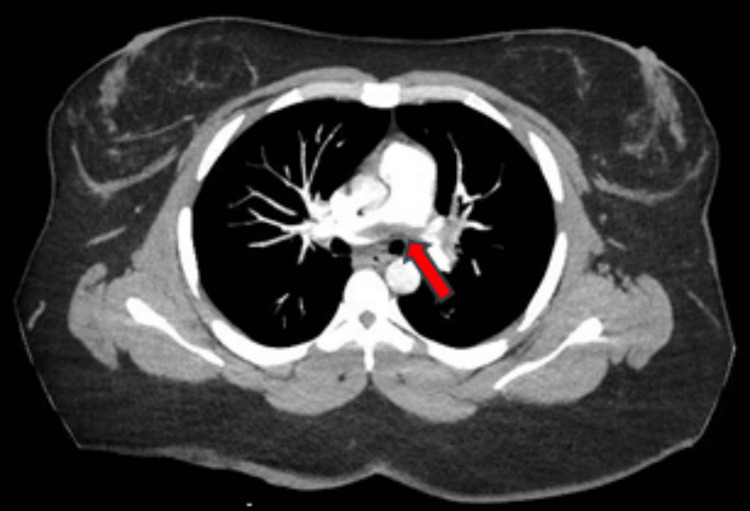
Initial CT angiogram from three months prior to admission showing extensive bilateral saddle pulmonary embolism (red arrow).
